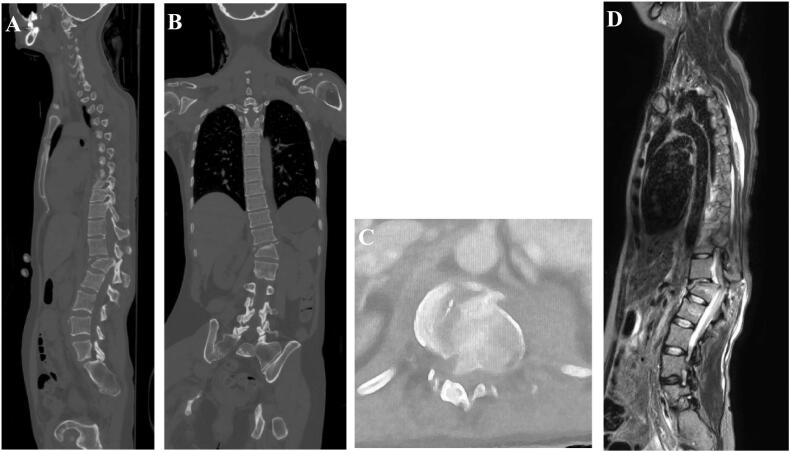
The sagittal, coronal reconstructions, and axial CT images demonstrated a fracture-dislocation at the Th12/L1 level (A–C). The MRI T2-weighted images of the whole spine revealed a fracture-dislocation at the Th12/L1 level, with significant compression of the dura mater (D).
